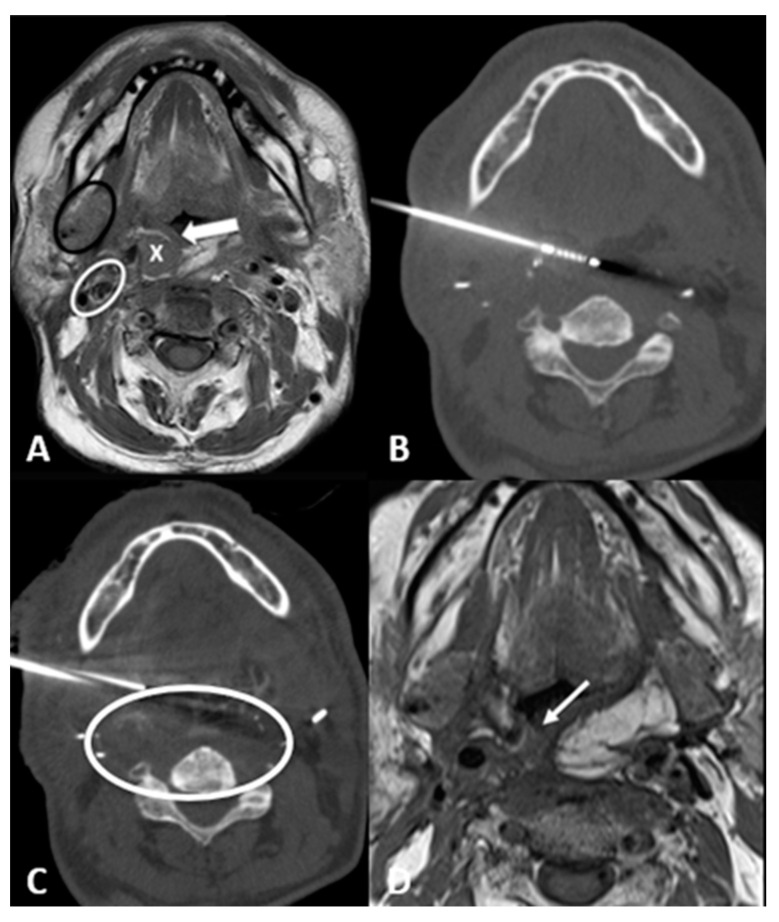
63-year-old male with recurrence of supraglottic squamocellular carcinoma which underwent previous total pharyngolaryngectomy and bilateral neck dissection. (A) T1-weighted unenhanced axial MRI scan showing the tumor recurrence (X), the mucosa of the neopharynx (arrow), the right submandibular gland (black circle), and the right carotid space (white circle). (B) Axial CT scan showing the cryoprobe positioned in the lesion through a trans-submandibular gland approach. (C) Axial CT scan showing parallel needle placement for hydrodissection of peritumoral tissue. (D) One-month T1-weighted unenhanced axial MRI scan showing the postablation tumor shrinkage (arrow).
